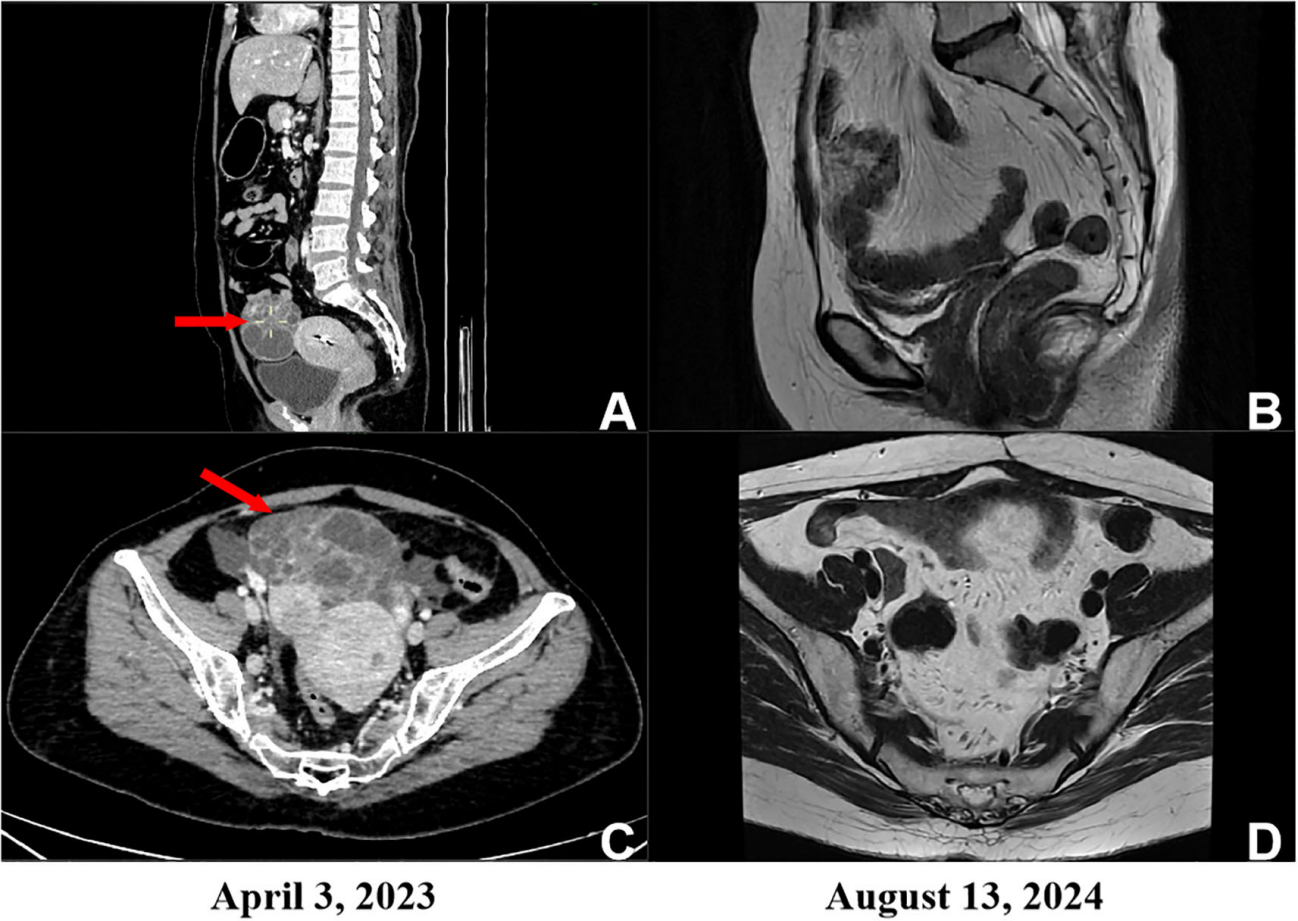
Pre- and post-operative pelvic imaging. (A, C) Preoperative coronal and axial CT images demonstrating a space-occupying lesion anterior to the uterus in the right pelvic cavity, highly suspicious for malignancy. (B, D) Corresponding coronal and axial MRI images obtained after comprehensive treatment, showing no radiologically detectable residual or recurrent disease.
